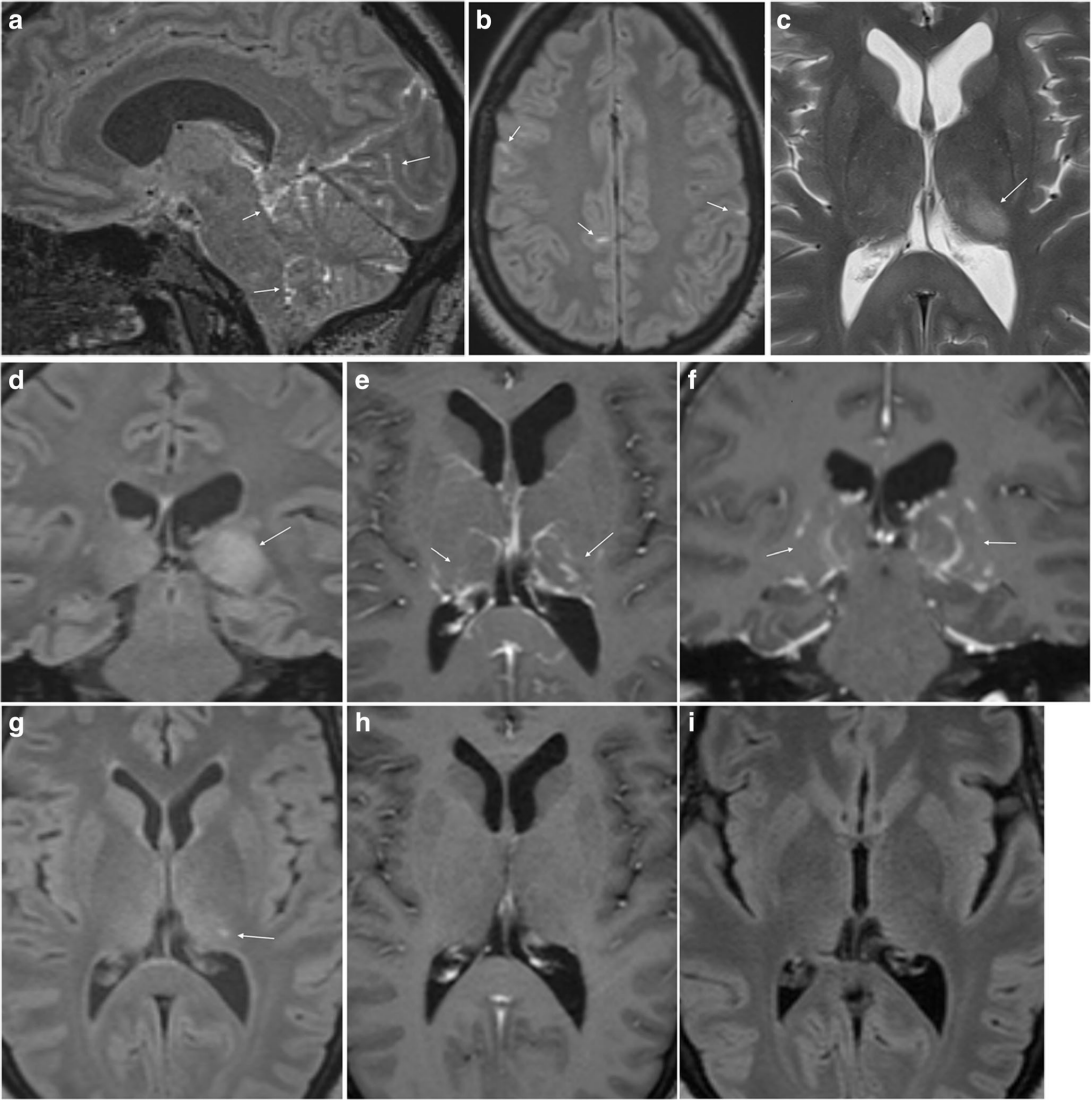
LNB meningoencephalitis in a 45-year-old female who presented with fatigue, headache, dizziness and vomiting. Neurological findings were tremor and unsteady gait. The CSF cell count was 270 cells/mm^3^ and there was a positive *Bb* IgG AI. Post-contrast sagittal (a) and axial (b) FLAIR images demonstrated patchy leptomeningeal enhancement over the cerebral convexities and in the posterior fossa (arrows). There was a hyperintense lesion in the left thalamus (arrow) on axial T2-weighted image (c) and coronal FLAIR image (d). There was no abnormality in the lesion on diffusion weighted images (not shown). Post-contrast axial (e) and coronal (f) T1-weighted images showed punctate and curvilinear uptake (arrows) in the thalamic lesion. Similar, subtle changes were present in the right thalamus. After 2 months there was only slight residual hyperintensity in the left thalamus (arrow) on axial FLAIR image (g) and no sign of enhancement on post-contrast axial T1 weighted image (h). One year after treatment there were no imaging abnormalities (i)
